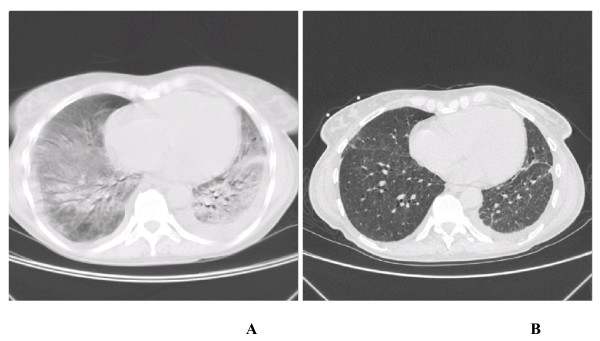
Selected scans from high resolution computed tomography before (A) and after (B) 15 days of treatment.
